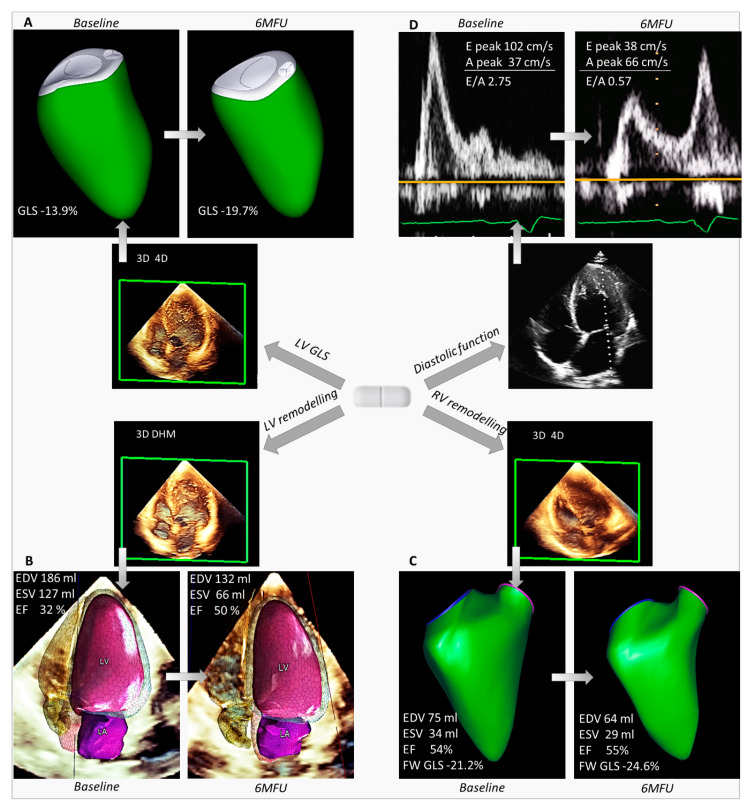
Evaluation of biventricular size and function by 3D transthoracic echocardiography and LV diastolic function by 2D Doppler parameters at baseline and 6-month follow-up after sacubitril/valsartan optimisation. Reported is the example of a patient with non-ischaemic HFrEF, showing significant improvement at 6MFU of (A) LV GLS, (B) LV volumes and LVEF, (C) RV volumes and function, and (D) diastolic function by 2D mitral valve inflow pattern. 6MFU = 6-month follow-up; EDV = end-diastolic volume; EF = ejection fraction; ESV = end-systolic volume; FW = free-wall; GLS = global longitudinal strain; LV = left ventricular; RV = right ventricular.
